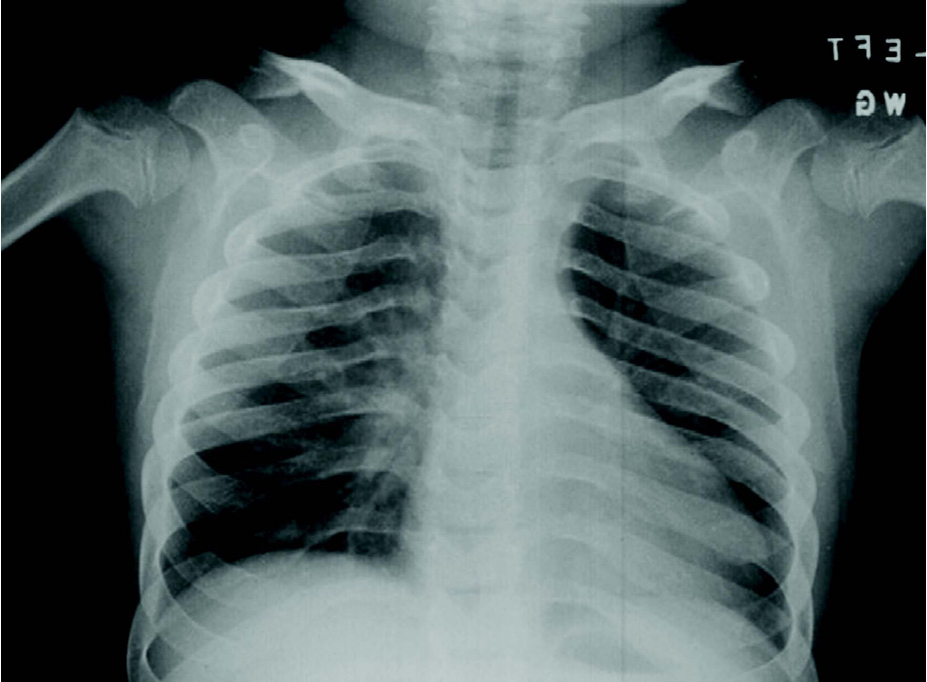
Rapidly progressing 12-year old male MPS VI patient: radiograph of clavicles and thorax.
